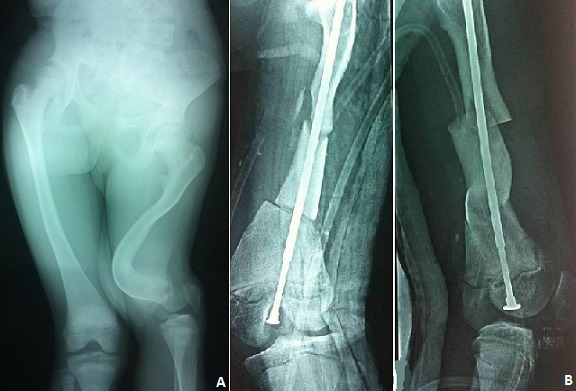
A) radiographie des deux cuisses objectivant une déformation historique du fémur, avec une angulation de 90°, épaississement des corticales et comblement du canal médullaire avec une coxa vara; B) résultat final après double ostéotomie de réaxation et enclouage centro-medullaire
